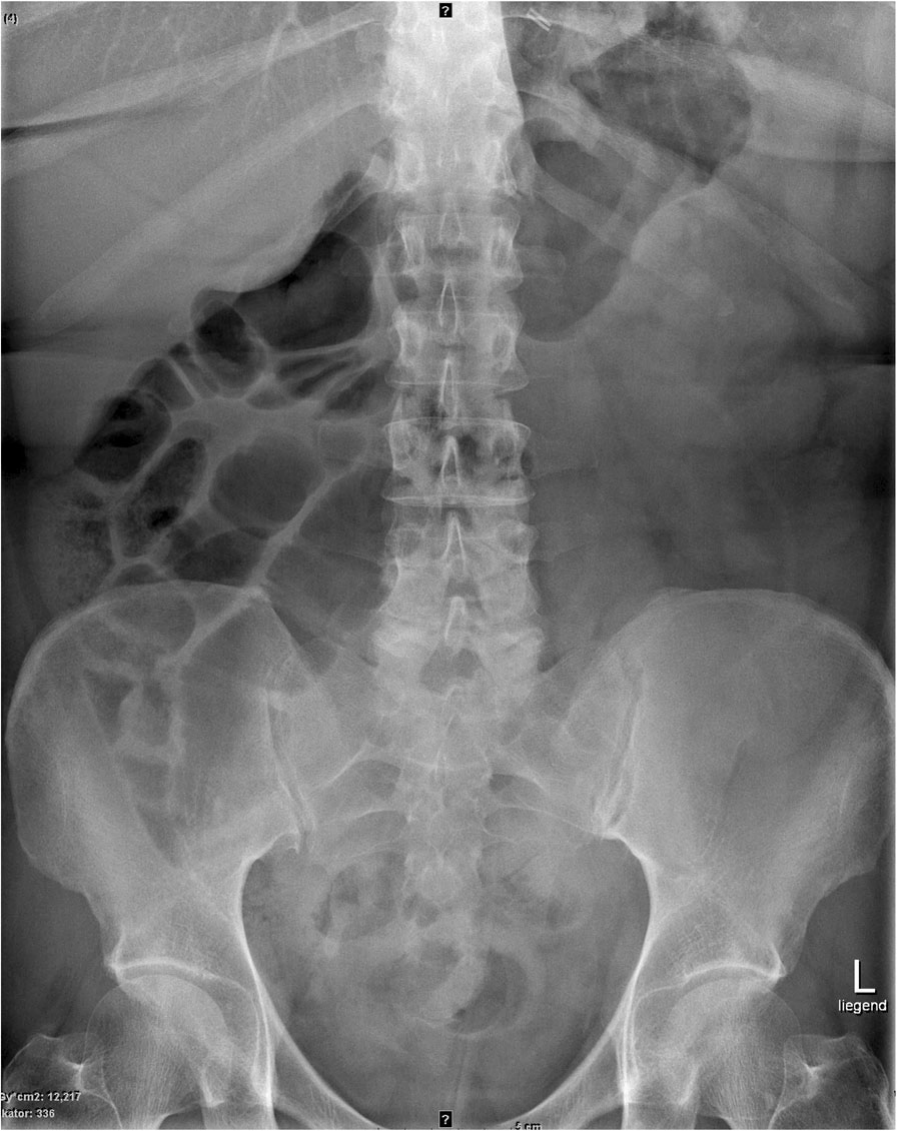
Plain X-ray showing dilated small bowel loops
